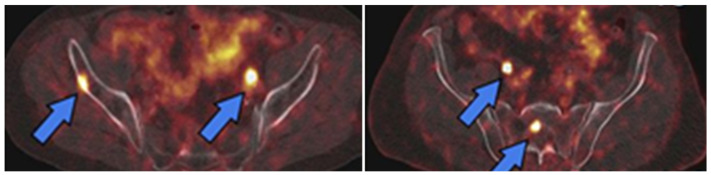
PET images of PSMA expression in PCa (blue arrows) using [^68^Ga]Ga-PSMA-11 (left image) and [^18^F]DCFPyL (right image). This research was originally published in the Journal of Nuclear Medicine (JNM) [61].
